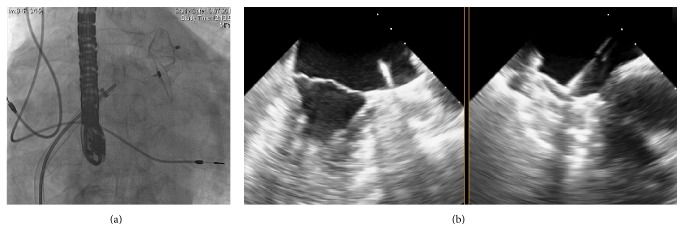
The implantation of the Amulet device was performed under fluoroscopic (a) and echocardiographic (b) guidance, similar to the Amplatzer Cardiac Plug (ACP).
